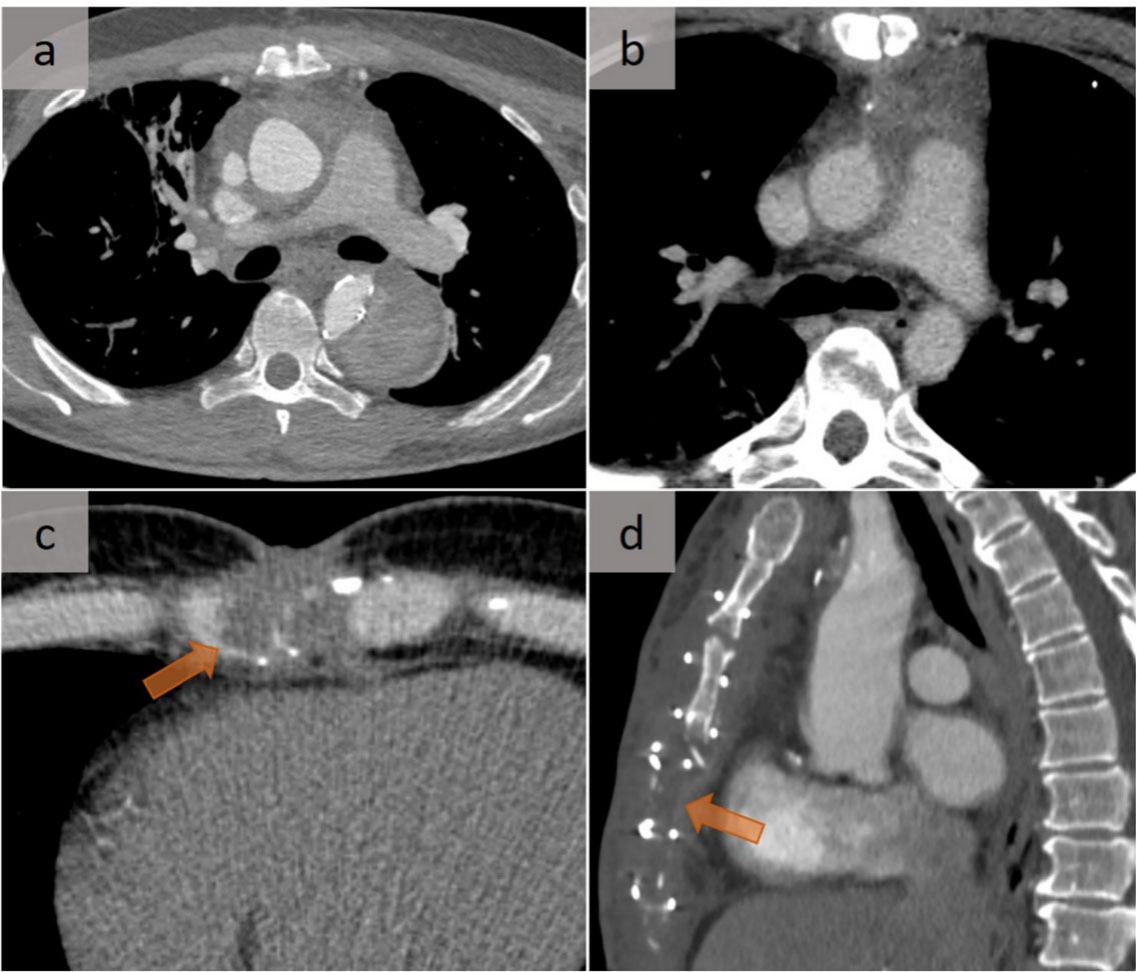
Post-operative changes versus mediastinitis. Similarities of CT aspects in two different conditions: normal post-operative appearance of the mediastinum during the first 2–3 weeks after Bentall surgery (a) and mediastinitis (b), both presenting as an increased attenuation of fat. In addition, the careful analysis of bone and cartilage, which is required in all cases of acute mediastinitis using bone-windowing, is well demonstrated in these two different cases with cartilage abscess (c) and sternal osteitis (d), respectively
